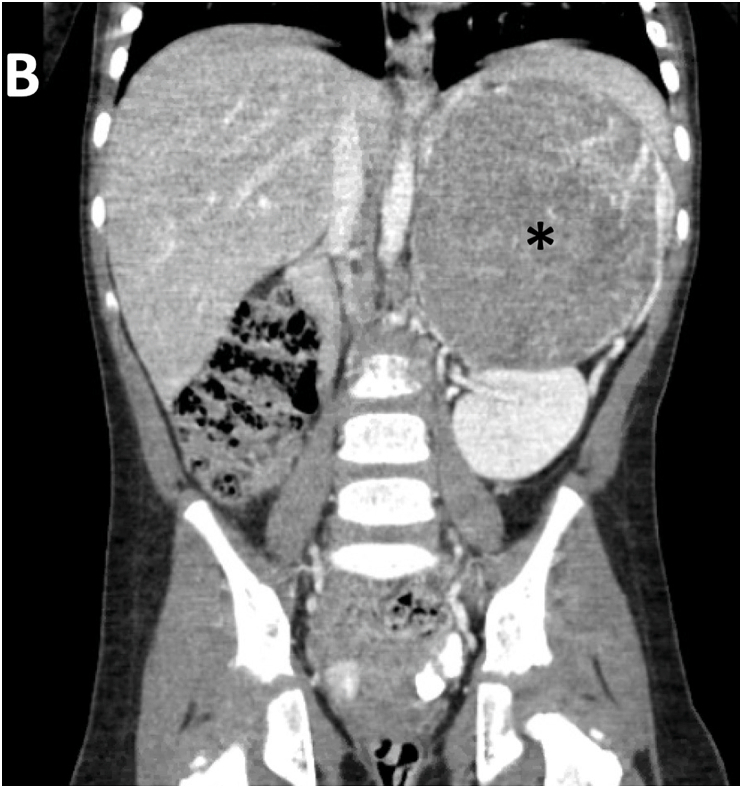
Computed tomography of abdomen. Contrast-enhanced imaging obtained at initial presentation identifying a large, well-circumscribed 9 cm left adrenal mass (*), just above the kidney.
